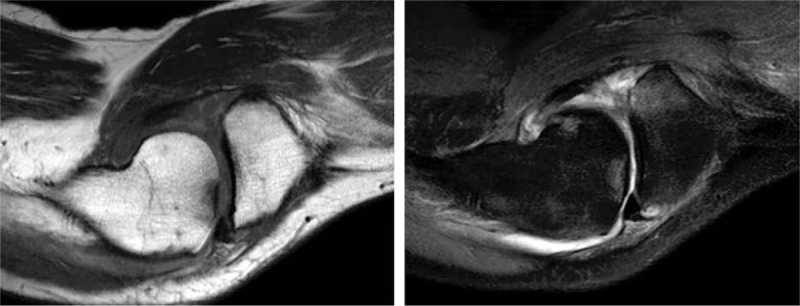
Magnetic resonance imaging (MRI) of the right knee showed bone marrow edema and synovitis.
